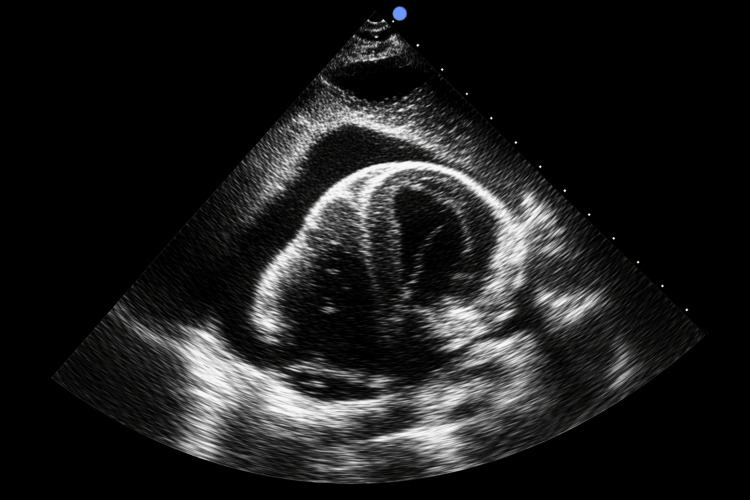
Additional echocardiography This view confirms a large pericardial effusion with tamponade physiology.
